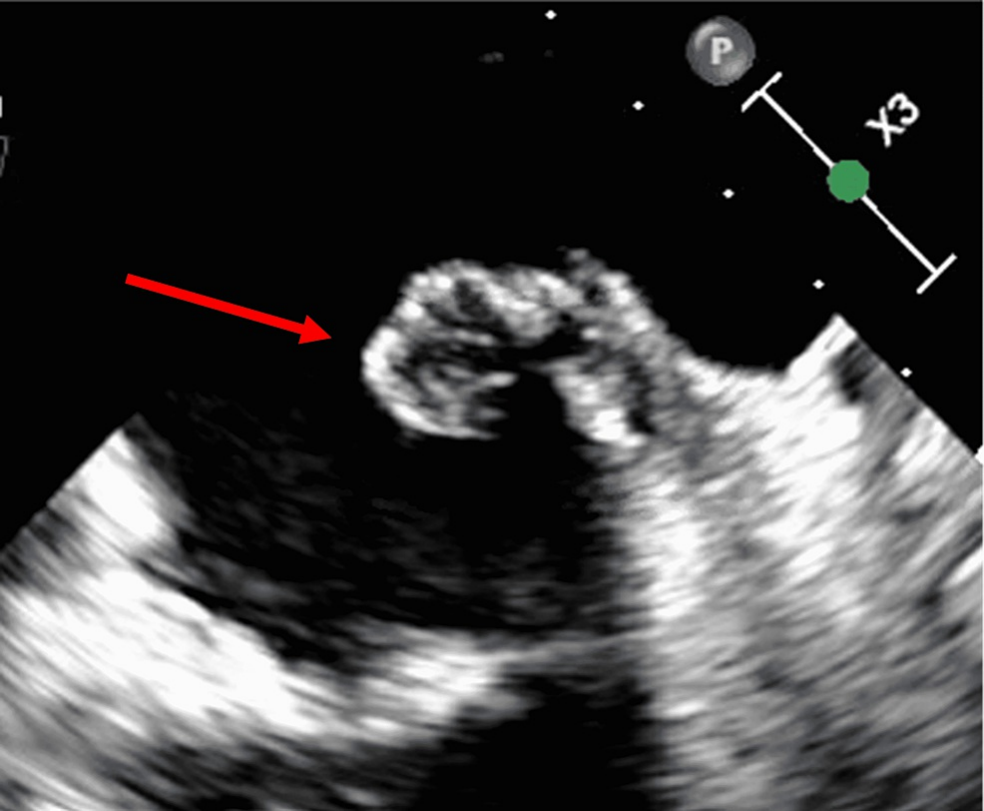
Transesophageal echocardiography (TEE) showing the largest myxoma, 2 cm in size (red arrow)
